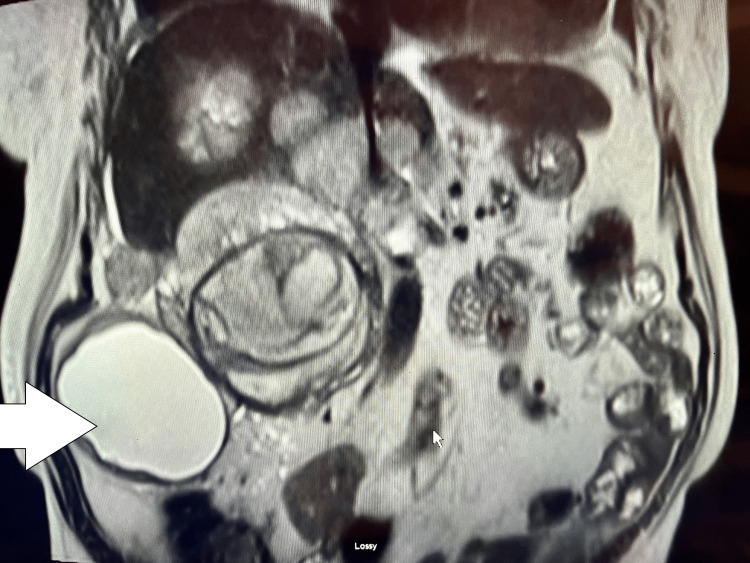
MRI image of the right retroperitoneal mass
